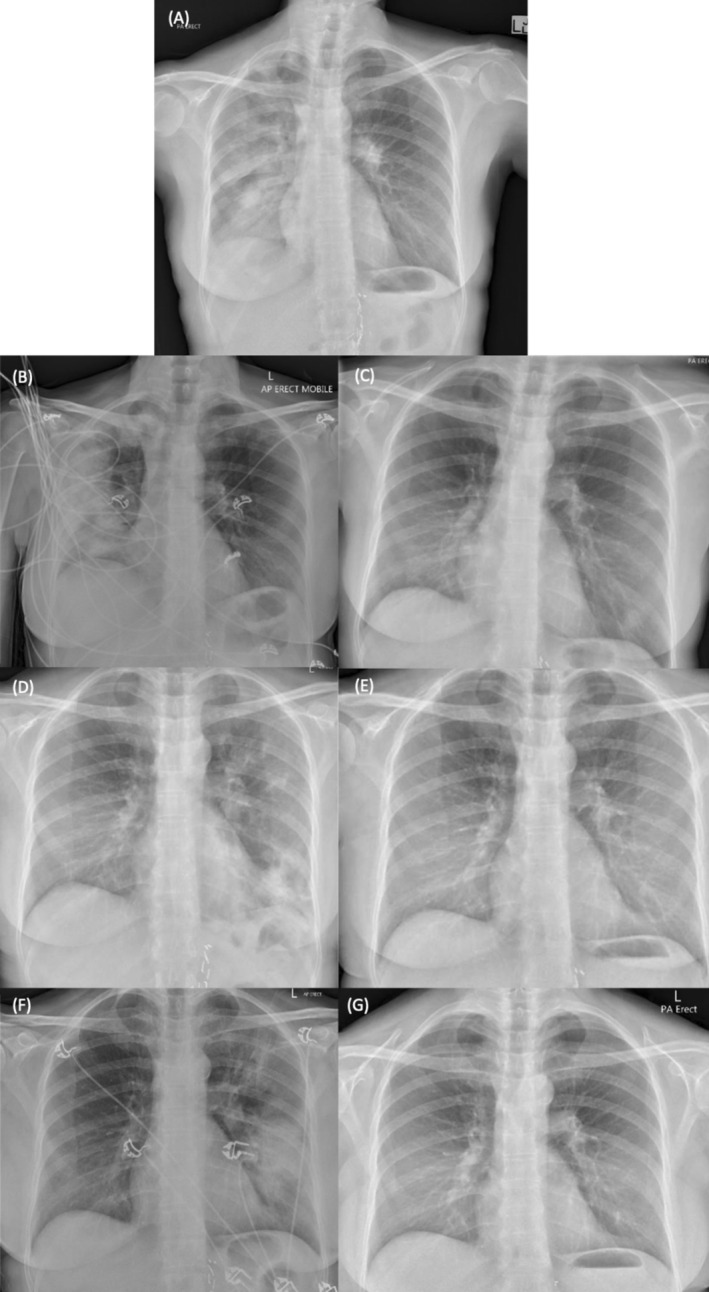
(A) Chest radiograph on initial admission demonstrating widespread consolidation throughout the right lung. (B) Radiograph obtained during the first recurrence revealing peripheral consolidation in the right lung. (C) Radiograph obtained after 3‐month prednisolone taper, showing resolution of the previous consolidation. (D) Radiograph obtained during the second recurrence showing irregular opacities in the left lower lobe. (E) Radiograph obtained after a 7‐month prednisolone taper, demonstrating resolution of the opacities. (F) Radiograph obtained during a recrudescence of disease 2 years later showing confluent opacities in the left mid‐zone and perihilar region of the left upper zone. (G) Radiograph obtained 4 months later during prednisolone tapering, showing resolution of opacities.
